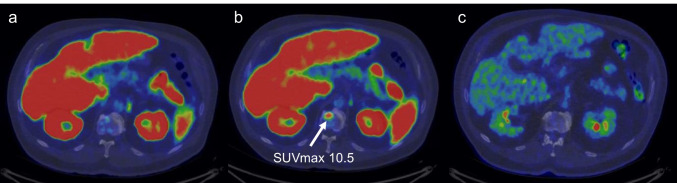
New PSMA bone uptake on L1 vertebra detected in the [^18^F] PSMA-1007 PET/CT after ADT. The uptake was not seen in the [^18^F]FDG PET/CT scan. a Baseline [^18^F] PSMA-1007 PET/CT. b [^18^F] PSMA-1007 PET/CT after ADT. c Baseline [^18^F]FDG PET/CT
